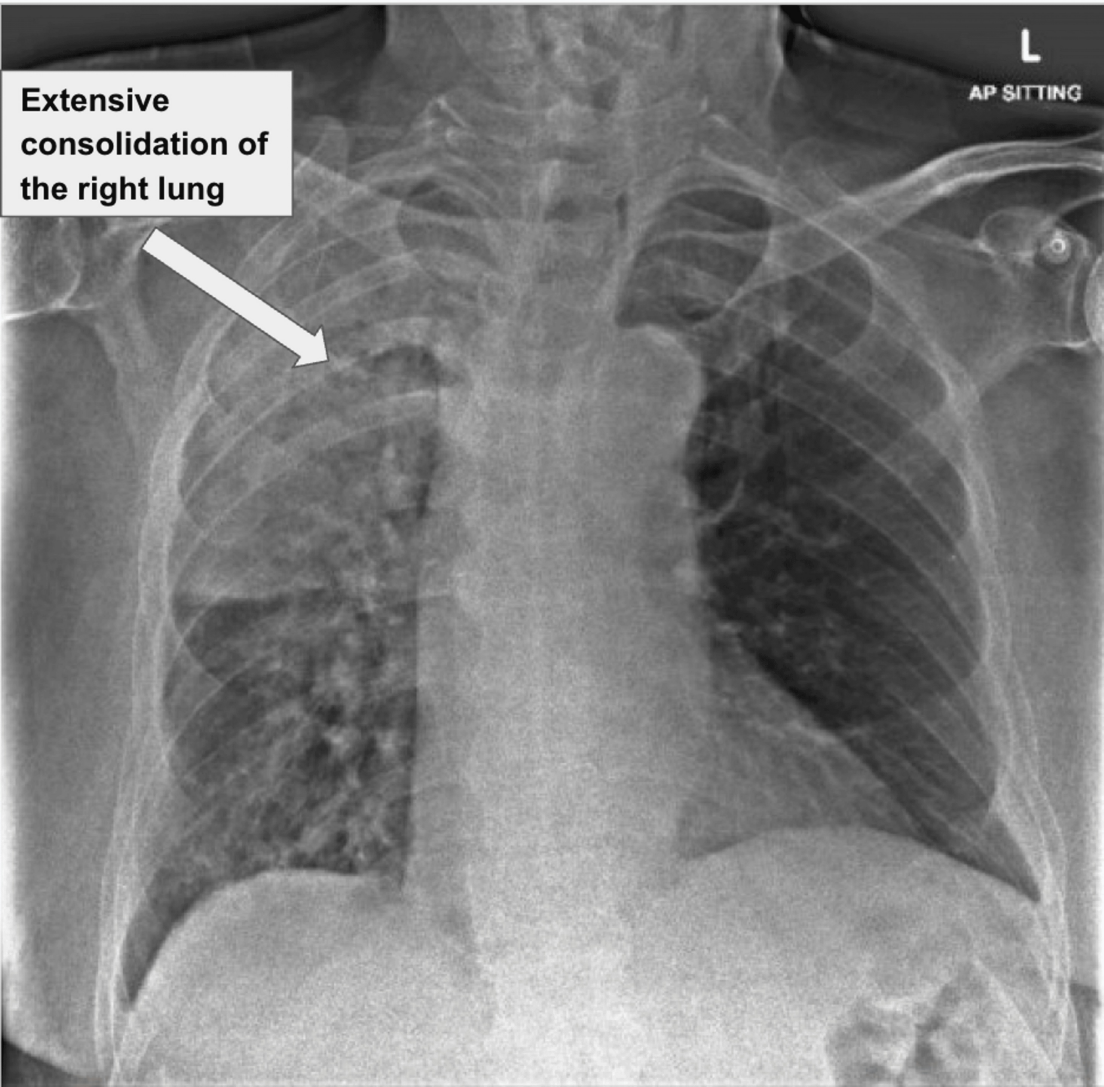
Chest radiograph demonstrating extensive consolidation of the right lung, characterized by widespread opacification and loss of normal aeration
